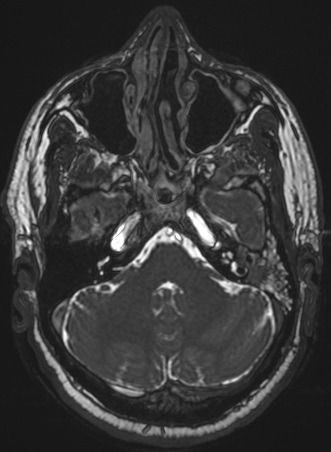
Magnetic resonance imaging (MRI) revealed a tissue process occupying the left middle ear in T2 isosignal
